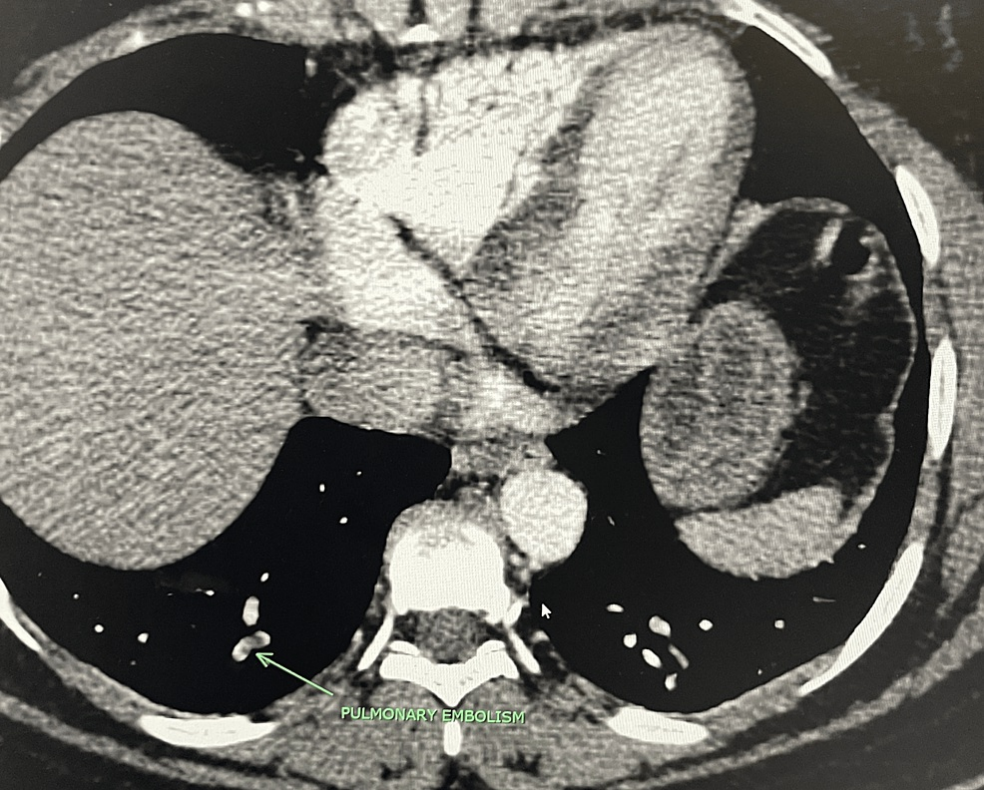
CT of the chest showing right-sided pulmonary emboli (arrow) CT: computed tomography
